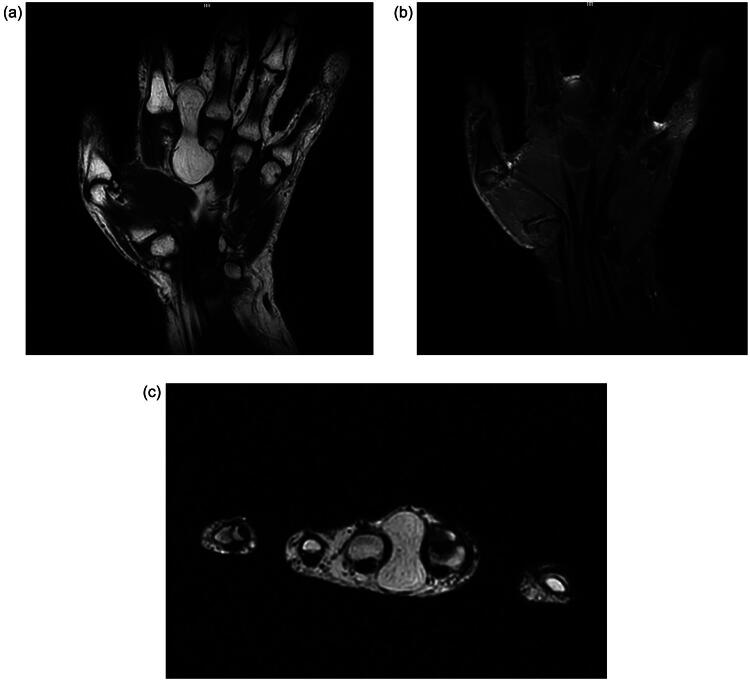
Magnetic Resonance Imaging (MRI) of the right hand demonstrating a well-defined, encapsulated soft tissue mass with homogeneous high signal intensity, consistent with a lipoma. (a) Coronal T1-weighted image shows a hyperintense, bilobed lesion located between the second and third metacarpophalangeal joints, consistent with adipose tissue. (b) Coronal STIR image confirms persistent high signal intensity without fat suppression, supporting the lipomatous nature of the lesion. (c) Axial T1-weighted image reveals the multicompartmental extension of the mass, displacing surrounding structures but without evidence of invasion.
